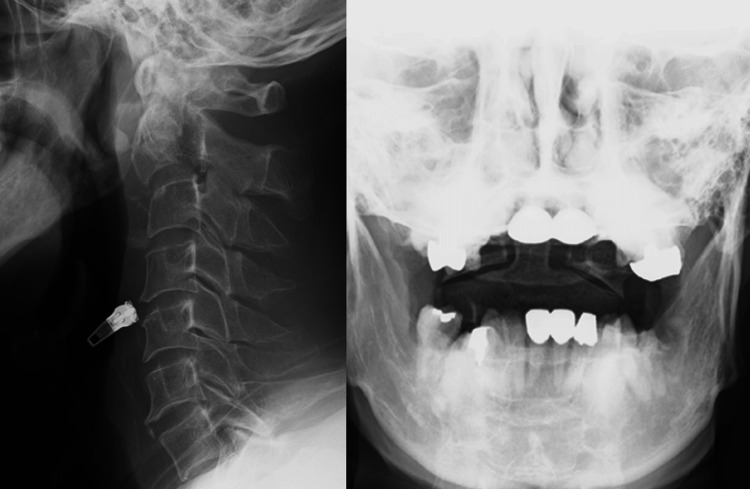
Preoperative plain X-ray images Displacement of C2 with angulation is observed. This fracture type is unstable.
